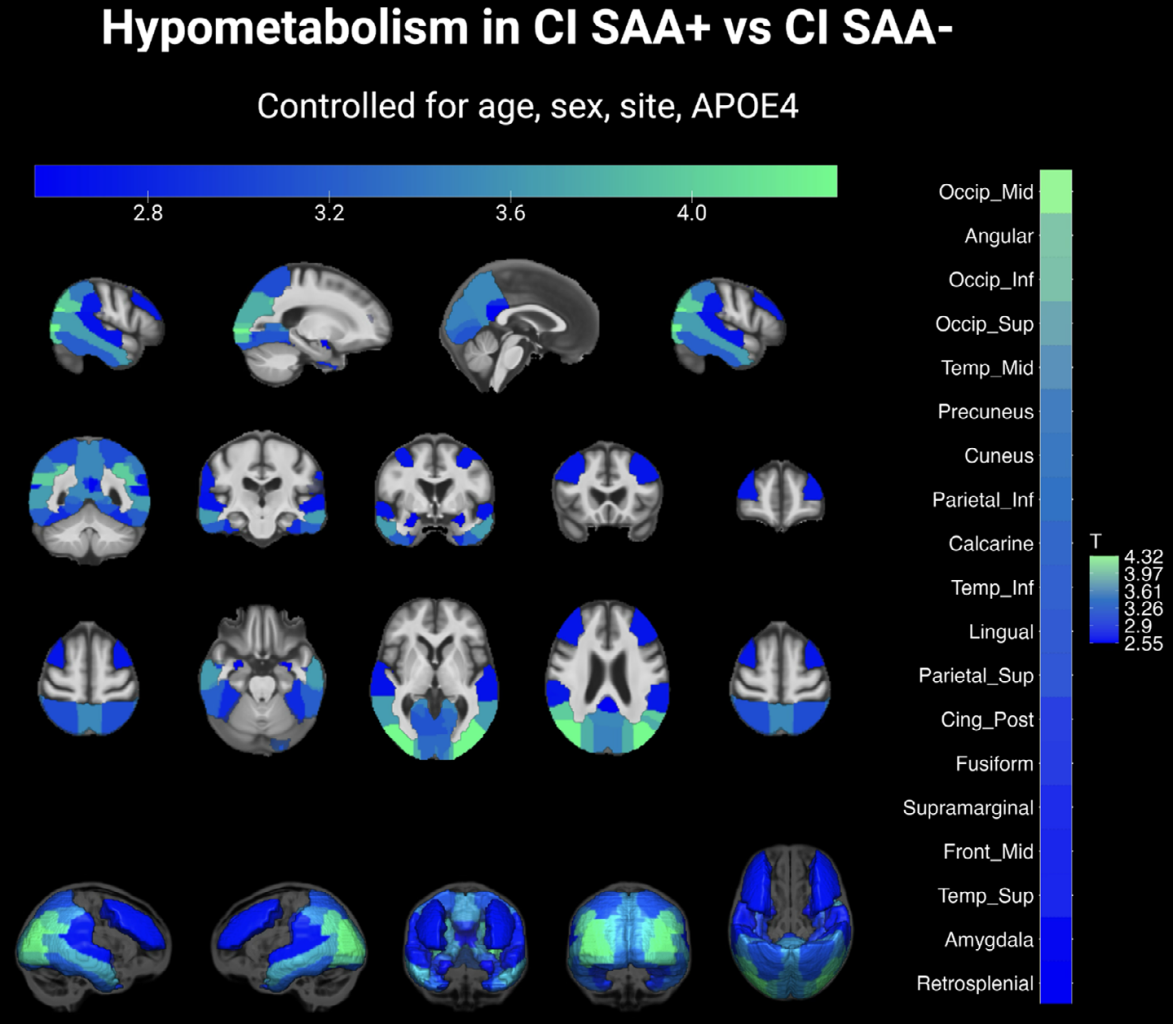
Regional FDG PET uptake comparison between α‐syn SAA+ and SAA− in CI individuals. *T*‐statistics are overlaid on the MCALT brain template, for regions where FDG uptake significantly differs between SAA+ and SAA− individuals (FDR corrected *q* < 0.05). Colors represent the *t* statistics ranging on an increasing scale from blue to green. Higher *t* statistics display a greater degree of hypometabolism in SAA+ individuals. Brain slices are shown in three orientations: sagittal (top row), coronal (middle row), and axial (bottom row). α‐syn, alpha‐synuclein; *APOE*, apolipoprotein E; CI, cognitively impaired; FDG PET, fluorodeoxyglucose positron emission tomography; FDR, false discovery rate; MCALT, Mayo Clinic adult lifespan template; SAA, seed amplification assay; SAA−, α‐syn seeding aggregates not detected; SAA+, α‐synuclein aggregates detected with an aggregation profile consistent with the characteristic seeding seen in Lewy body diseases
